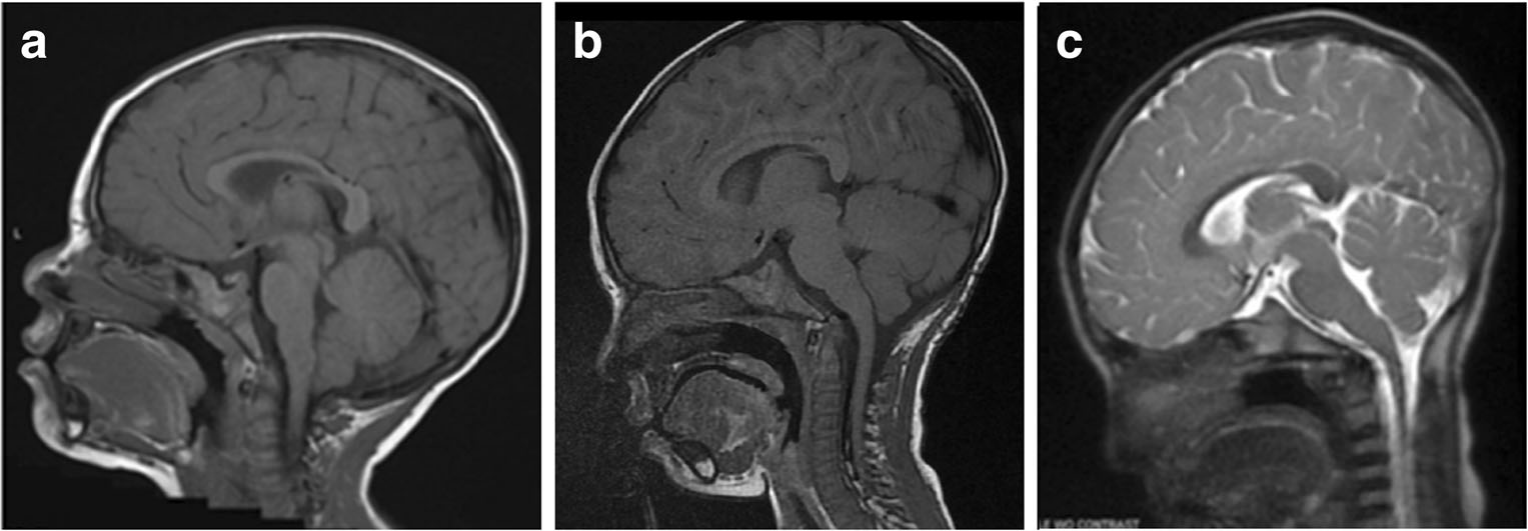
A 20-month-old boy (Case 5) with a 3-month history of increasing emesis and grabbing his occiput. Preoperative midsagittal T1-weighted MRI (A) showed Chiari I malformation with tonsils herniating up to above the C1. He had a posterior fossa decompression with dural scoring (Type 1 procedure). Postoperatively, all symptoms resolved, andwith improved decompression site shown on midsagittal T1-weighted MR (B) obtained 4 months postoperatively. A 3-year postoperative MR showed spontaneous improvement of the decompression site (C).
